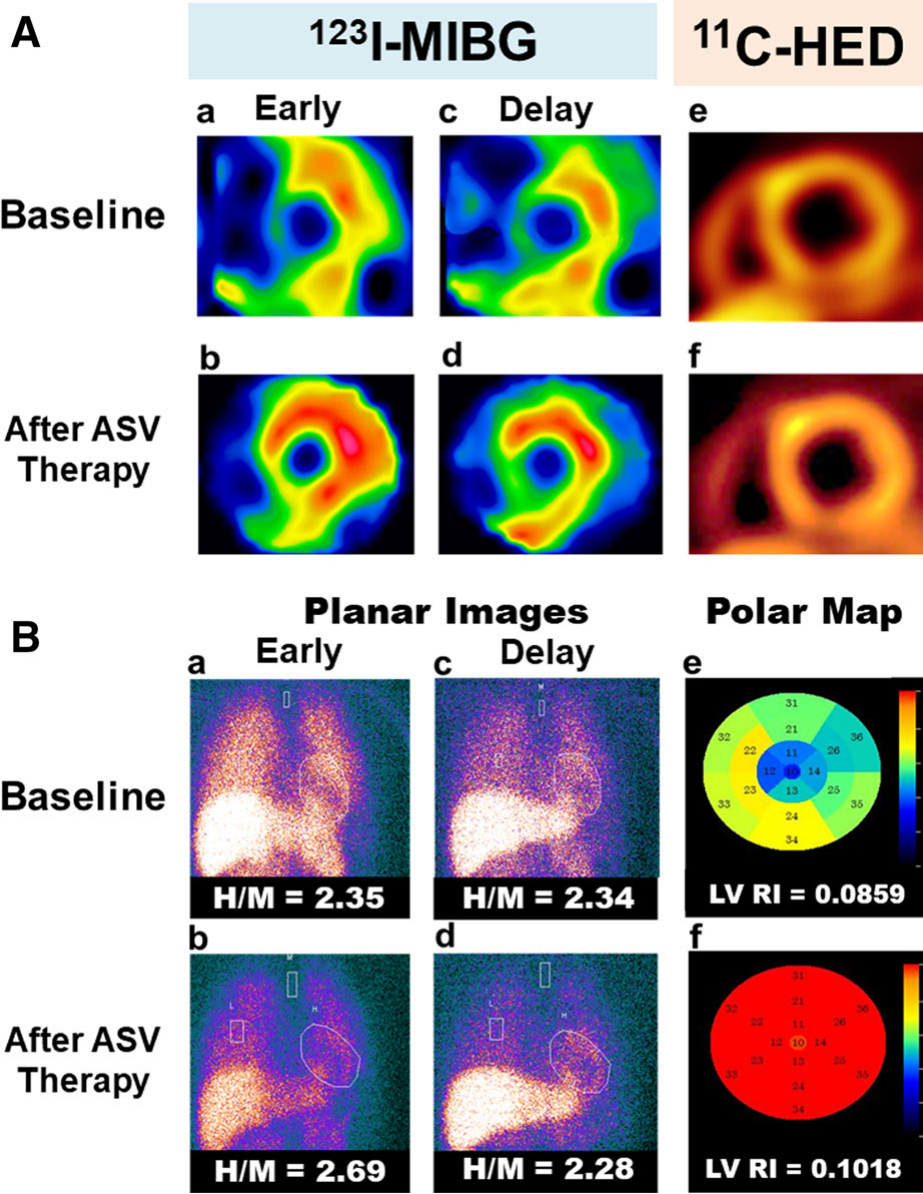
Representative images of 27-year-old female with ^123^I-MIBG planar/SPECT and ^11^C-HED PET/CT. A Short-axis images of ^123^I-MIBG SPECT and ^11^C-HED PET/CT. B Planar images of ^123^I-MIBG and polar map images of ^11^C-HED PET/CT. a Early ^123^I-MIBG SPECT images at baseline. b^123^I-MIBG SPECT images after 6 months of ASV. c Delayed ^123^I-MIBG SPECT images at baseline. d Delayed ^123^I-MIBG SPECT images after 6 months of ASV. e^11^C-HED PET/CT images at baseline. f^11^C-HED PET images after 6 months of ASV. Representative case of 27-year-old female with heart failure with improved AHI (15.6 to 0.5/h) and LVEF (38.0 to 45.0%) after ASV therapy. Global ^11^C-HED PET/CT RI, a presynaptic function marker of SNF, significantly improved after ASV treatment (Figure 1B-e, 1B-e at baseline; and 1B-f after ASV). The early H/M ratio also increased (Figure 1B-a at baseline; and 1B-b after ASV), whereas the delayed H/M ratio, a turnover parameter of SNF, did not change (Figure 1B-c at baseline; and 1B-d after ASV). *ASV*, adaptive servo-ventilation; *HED*, ^11^C-hydroxyephedrine; ^*123*^*I-MIBG SPECT*, ^123^I-metaiodobenzylguanidine single-photon emission computed tomography; *PET*, positron emission tomography; *H/M*, heart/mediastinum; *RI*, retention index; *SNF*, sympathetic nervous function; *LVEF*, left ventricular ejection fraction
